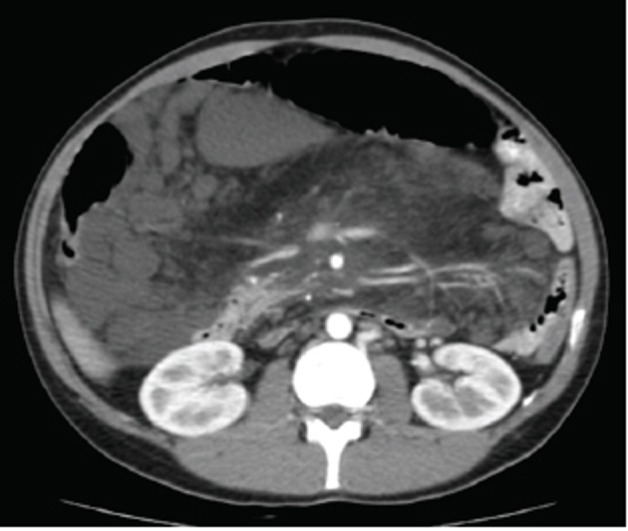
CT scan showed an abdominal mass extended from the hepatic hilus to the pelvis; it was composed of fatty areas (−85HU) near the mesentery and a hyperdense (32HU) portion located dorsally.
